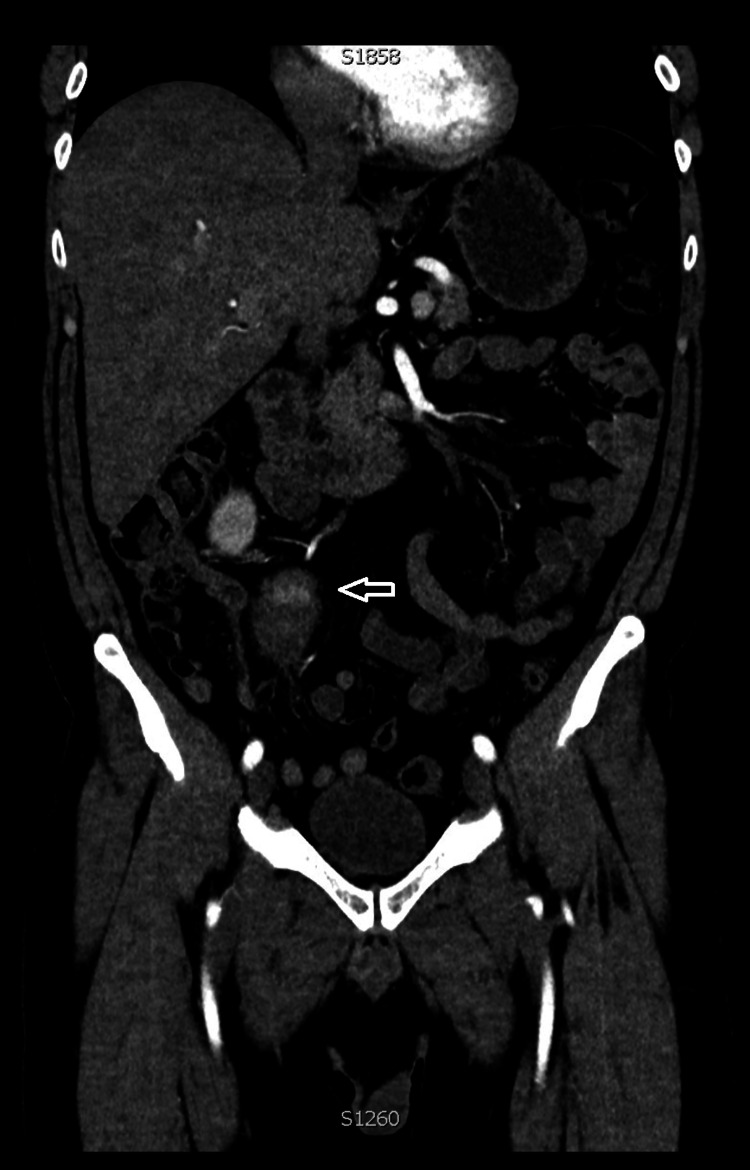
Coronal image of CT abdominal angiogram which shows the early enhancing pseudoaneurysm in the right iliac fossa (arrow).
